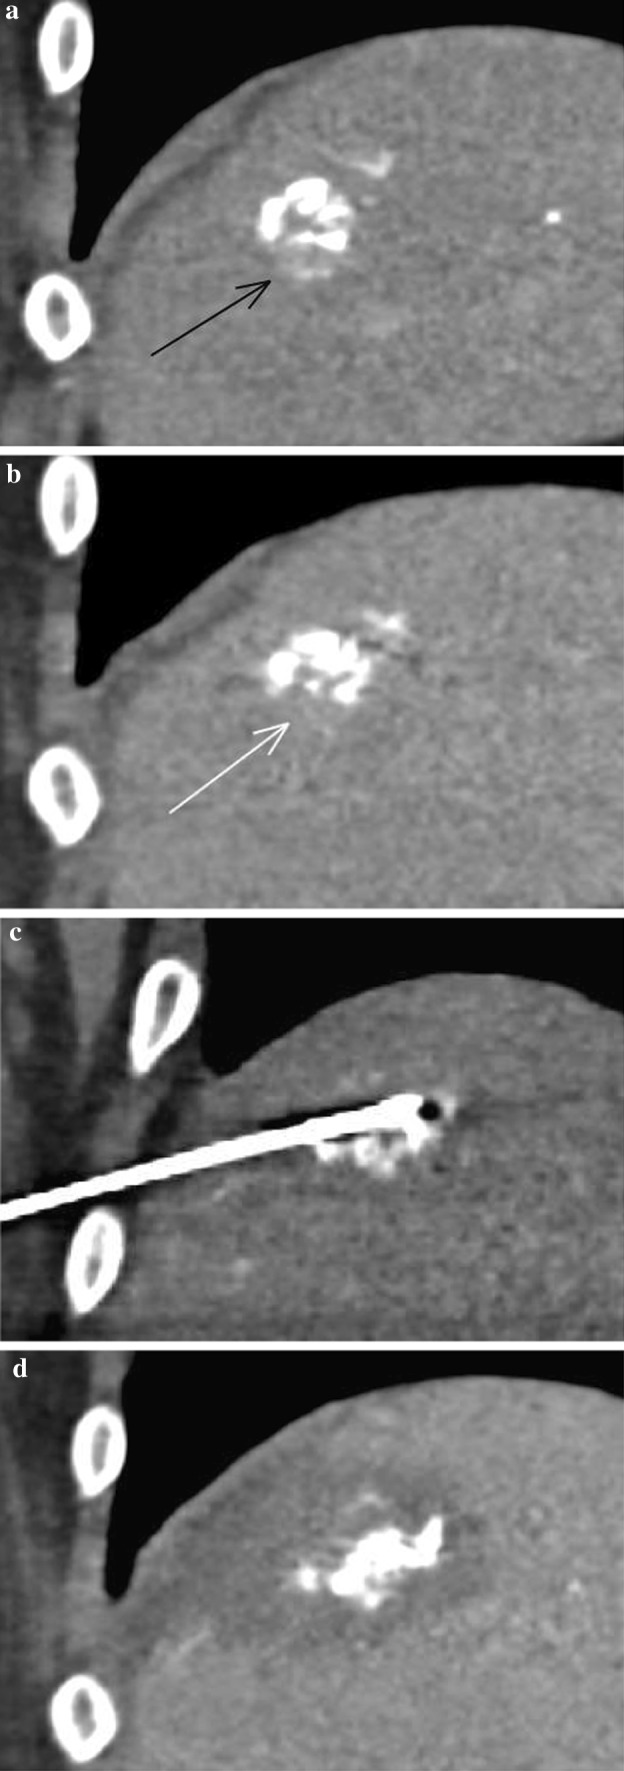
CT-guided ablation following TACE. Figure 3a, a coronal image from an arterial phase scan 18 months after the initial TACE, shows a small nodule of enhancement (black arrow) at the inferior aspect of the previously treated lesion, with washout on the venous phase (Fig. 3b, white arrow). The radiopaque beads allowed the lesion to be targeted for thermal ablation under CT guidance—Fig. 3c shows the microwave needle in the lesion. Figure 3d is from a venous phase scan one month later, showing satisfactory ablation margins around the radiopaque beads in the lesion
